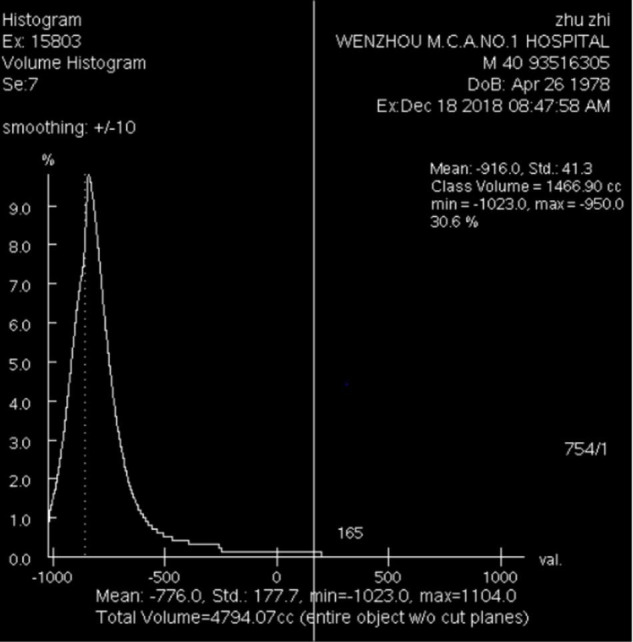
Emphysema volume, total lung volume, emphysema index and MLDins of the entire lung on inspiration CT scan (–950 HUs).
